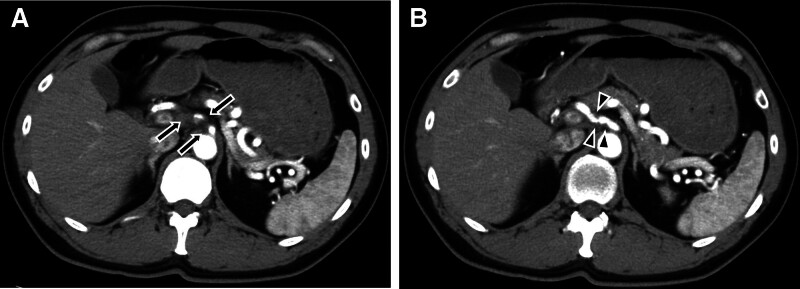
Two pictures of abdominal computed tomography angiography (A and B). The splenic artery shows high tortuousness. Slight wall thickening (arrows) and irregularly narrowed lumen (arrowheads) are noted at the celiac trunk, extending to the common hepatic artery and left gastric artery.
